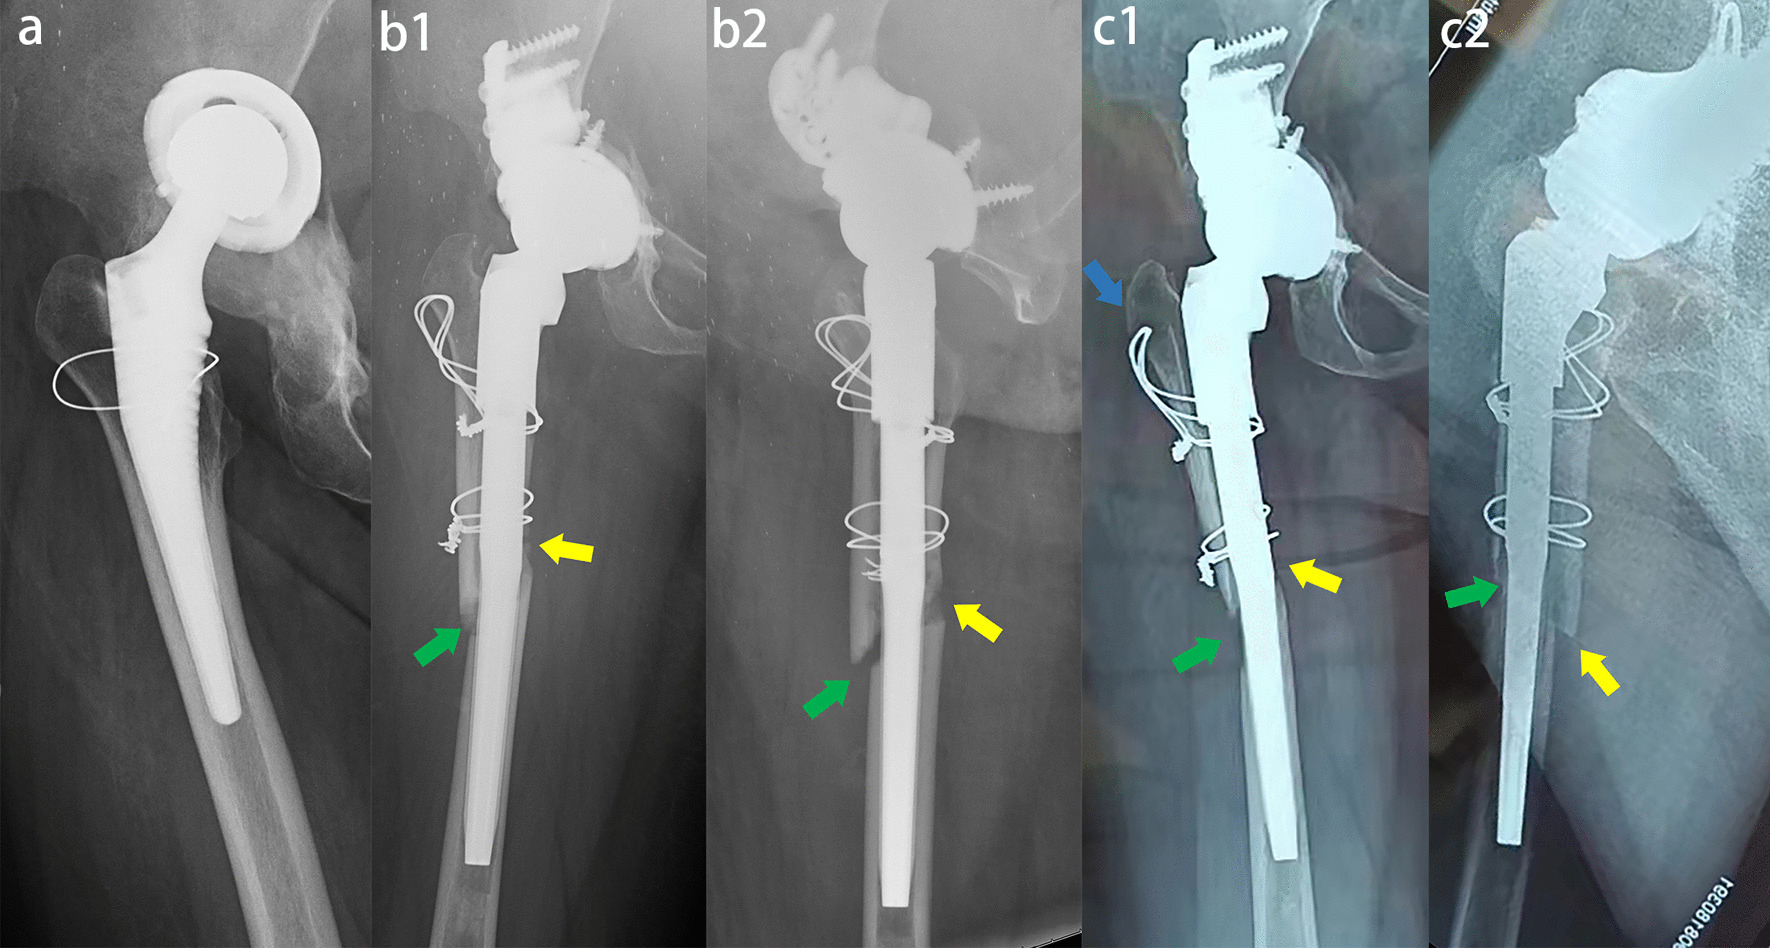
Female, 43 years, aseptic loosening. a Preoperative X-ray showed that the stem was well fixed and was removed by using ETO. b Anteroposterior and lateral X-rays immediately after operation (b1 and b2). MP stem was used to treat with intraoperative medial femoral cortical fracture (yellow arrow). X-ray showed nonunion of the ETO osteotomy fragment (green arrow). c Anteroposterior and lateral X-rays two years after operation (c1 and c2) showed bone atrophy in osteotomy line (green arrow). The medial cortical fragment was nonunion and migrated (yellow arrow). Osteopenia of medial cortical bone due to fracture and loosening
